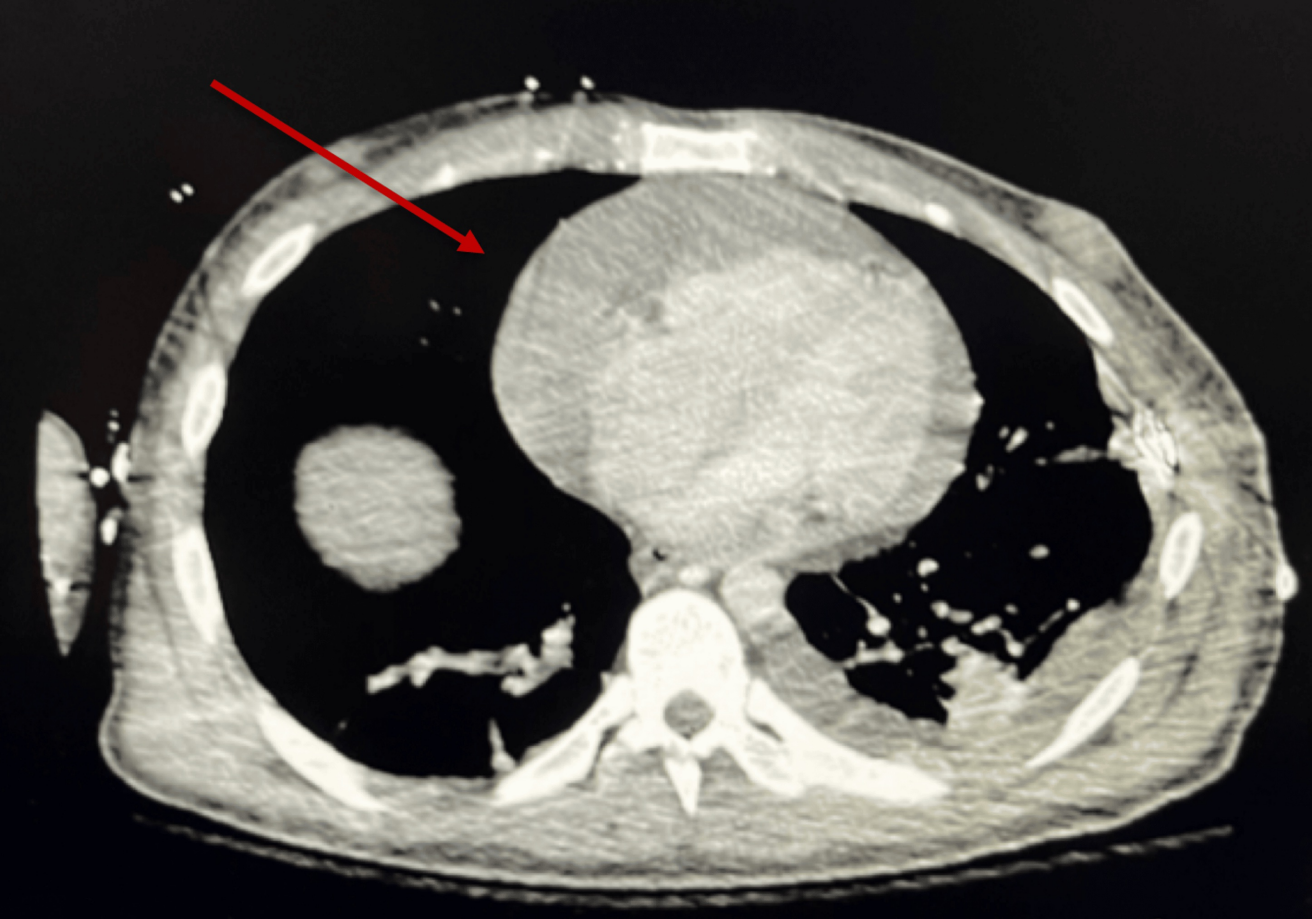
Chest CT 15 days after the initial trauma showing massive pericardial effusion The red arrow shows the accumulation of fluid within the pericardial sac.
